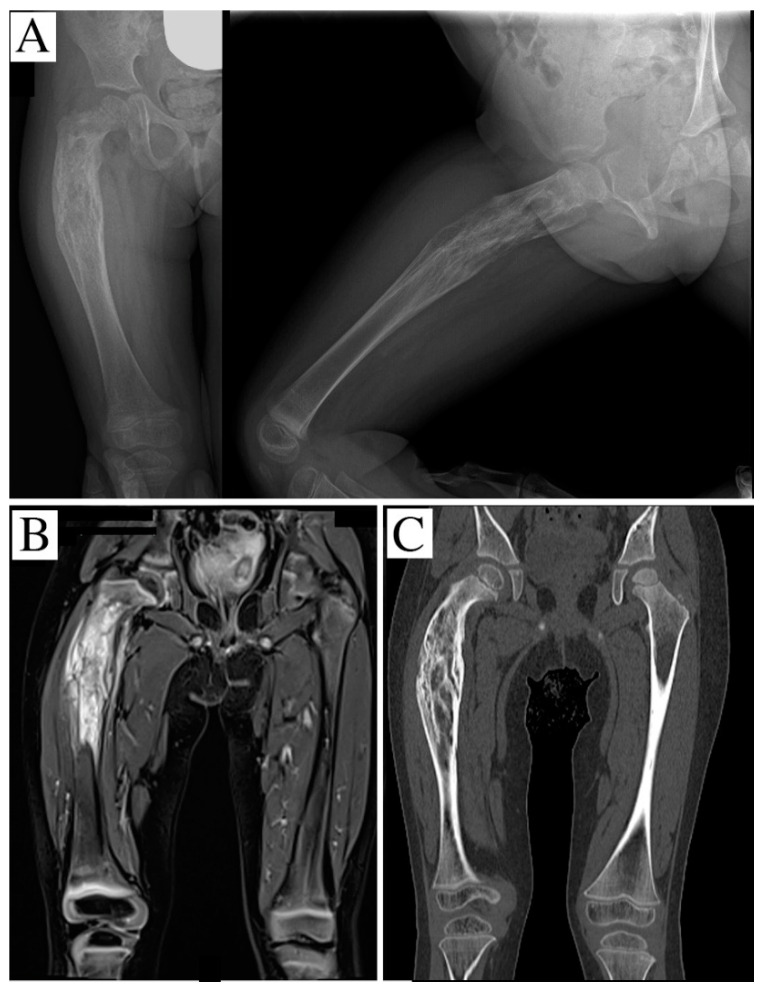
(A) an X-ray showing an osteolytic and apparently well-defined lesion of the proximal and middle third of the right femur; the segment is bent with onion-skin periosteal reaction; (B) STIR-weighted coronal-MRI reconstruction, evidencing permeative behavior and an intense edema. (C) CT scan showing the geographic osteolysis.
